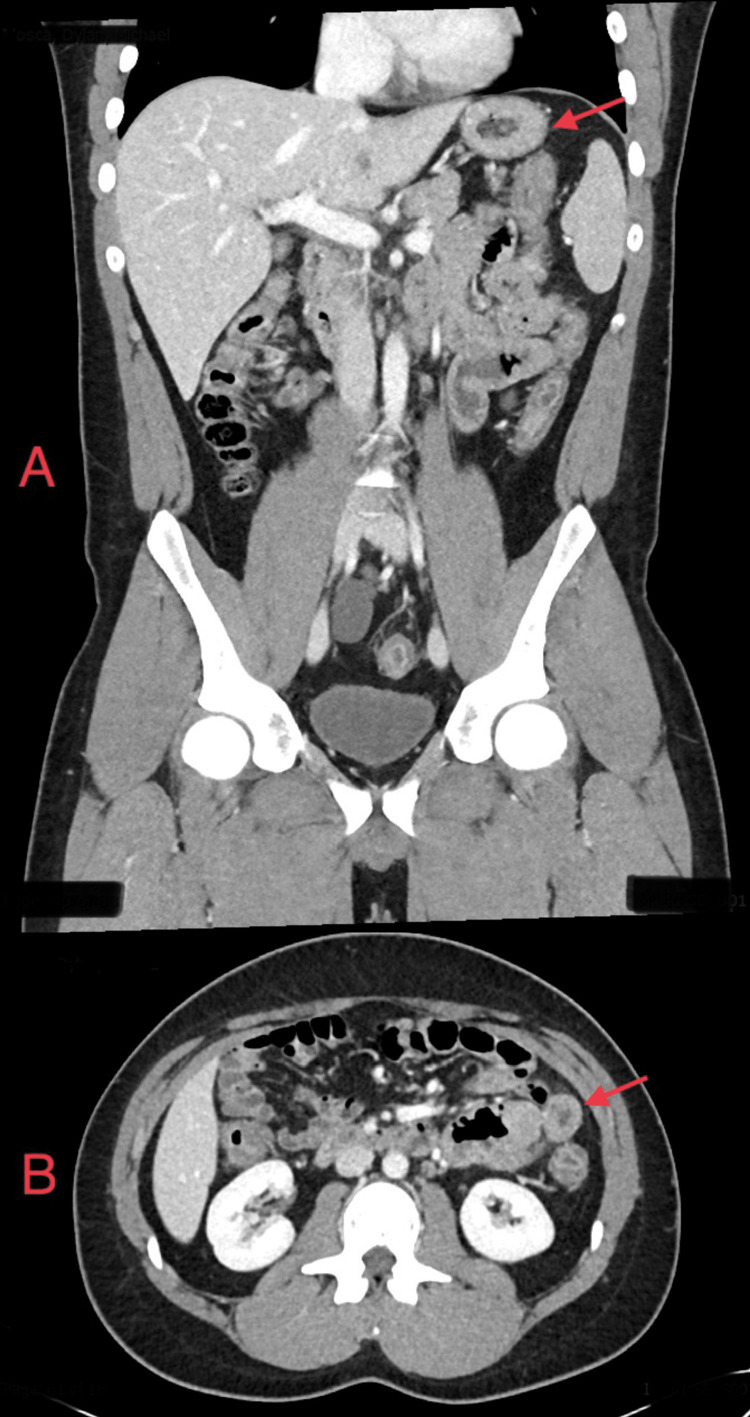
CT abdomen shows circumferential wall thickening of the colon (red arrows).
